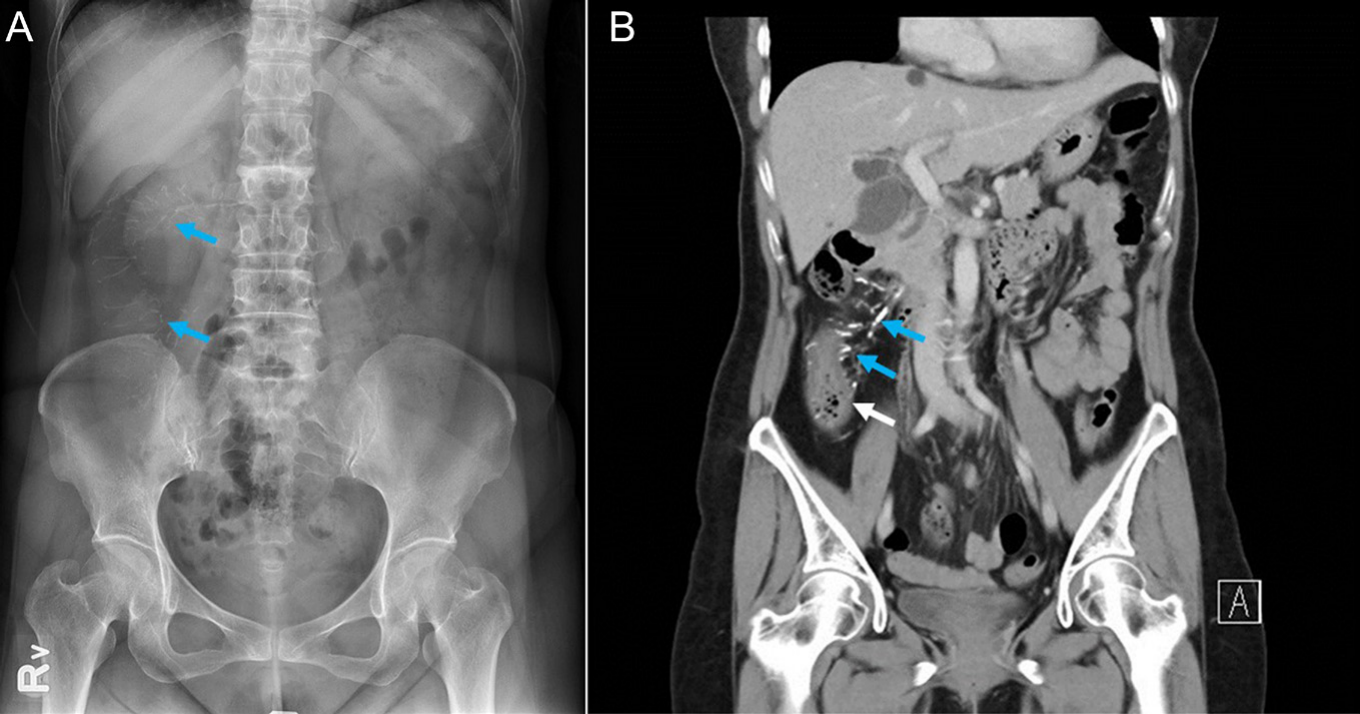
(A) Pelvic X-ray showed thread-like calcifications at ascending colon (blue arrows). (B) Multiple calcifications (blue arrows) are depicted along the thickened wall of ascending colon (white arrow).
